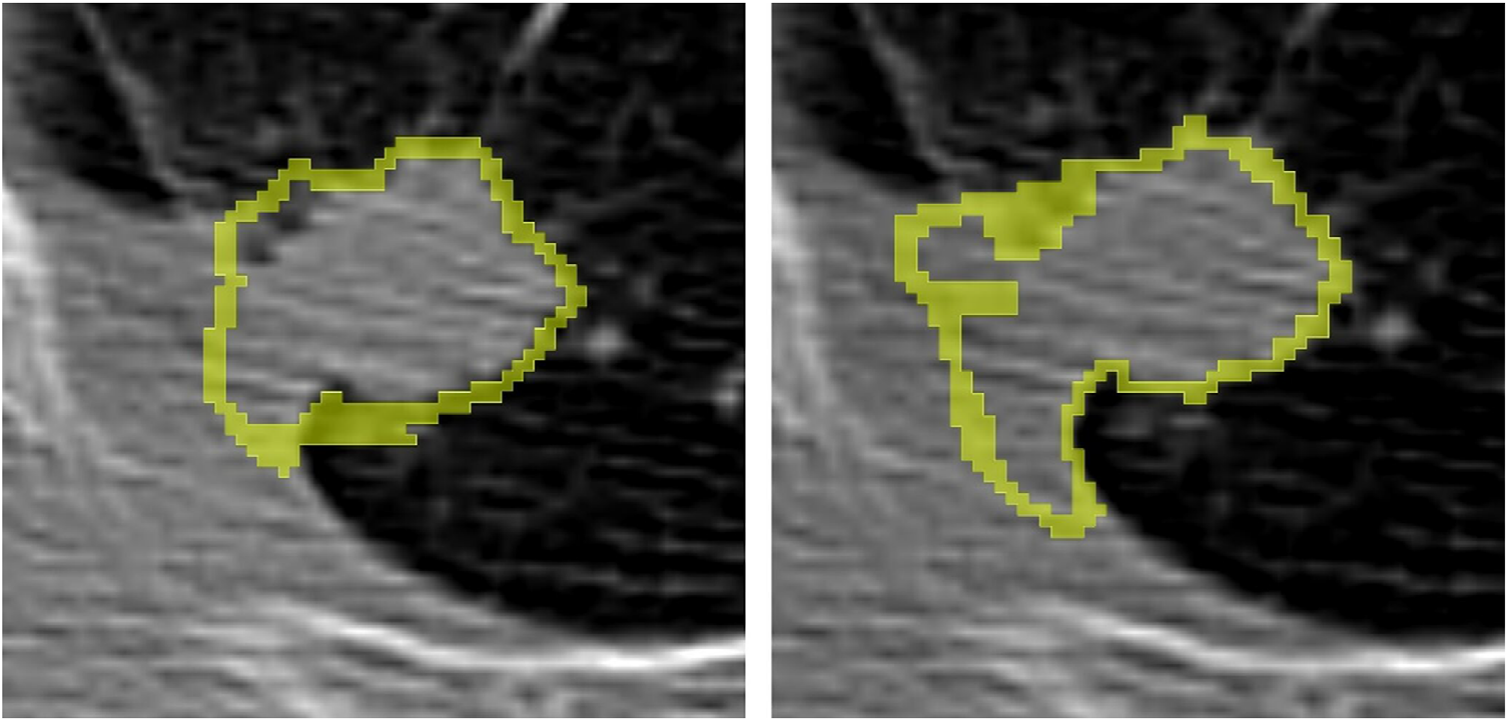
Two contours on the same CT image set of one patient are different.
